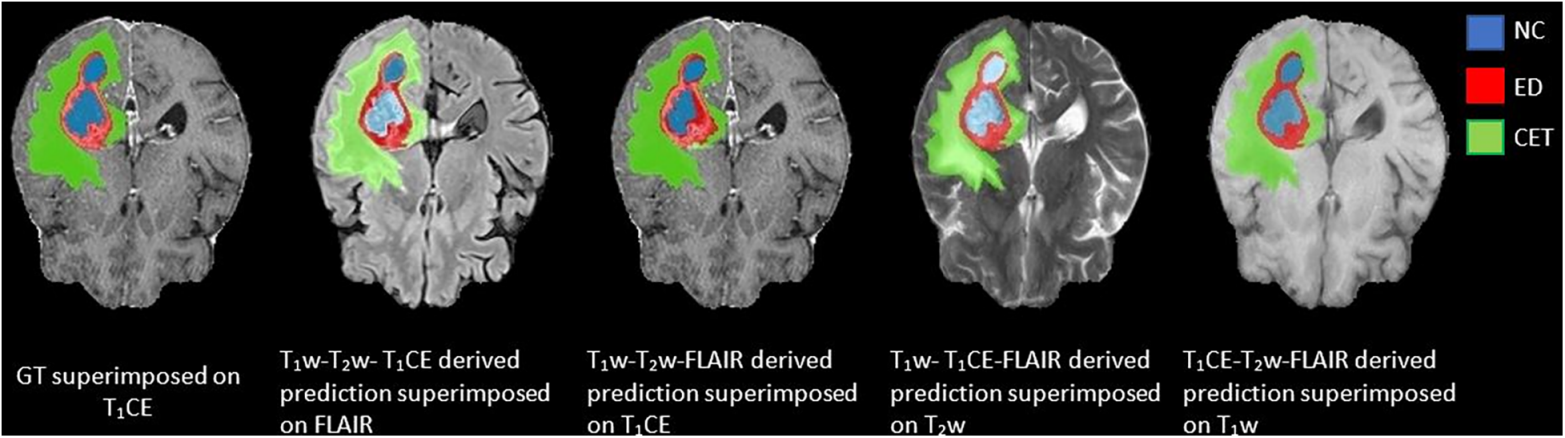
Predictions generated using a three-input trained DeepMedic model with original image combinations of T_1_CE–T_1_w–T_2_w, T_1_w–T_2_w–FLAIR, T_1_CE–T_1_w–FLAIR, and T_1_CE–T_2_w–FLAIR superimposed on missing input, presented with GT superimposed on T_1_CE image for comparison.
